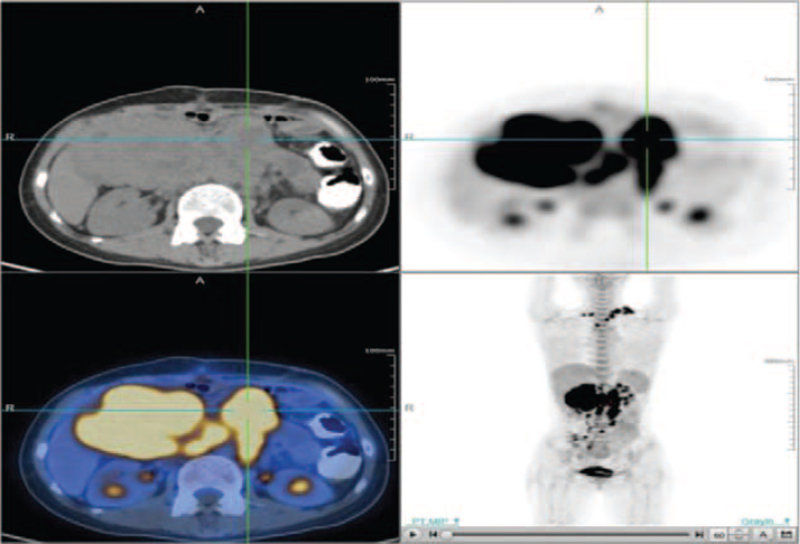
PET/CT showed a large duodenal mass with strong FDG uptake and multiple lymph node metastases.
